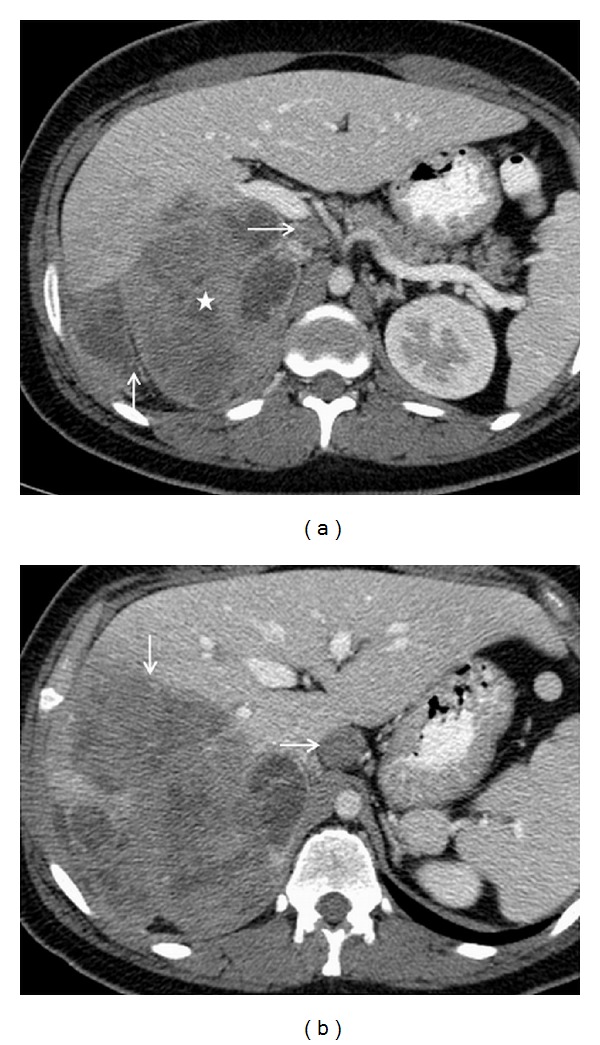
(a) Axial contrast-enhanced multidetector computed tomography (MDCT) image of the upper abdomen reveals a large mass of heterogeneous attenuation replacing the midportion and superior pole of the right kidney (star). Mass has broken into the hepatorenal space (vertical arrow) and invaded the right liver. A metastatic pericaval lymph node is seen (horizontal arrow). (b) Axial contrast-enhanced MDCT image shows a large lobulated component of the renal mass invading the right liver (horizontal arrow). An enlarged metastatic left gastric lymph node is also noted (vertical arrow).
